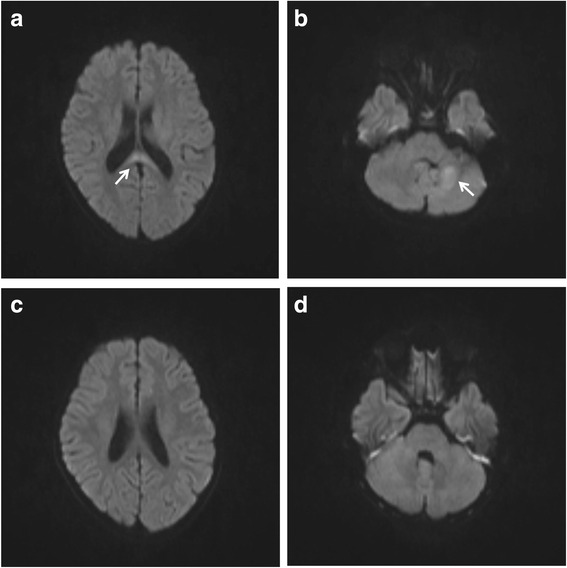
The brain MRI in case 2. The brain MRI on day 5 revealed high intensity lesions (arrows) in the SCC (a) and the left cerebellum (b) on diffusion-weighted images, which disappeared on day 12 (c and d)
